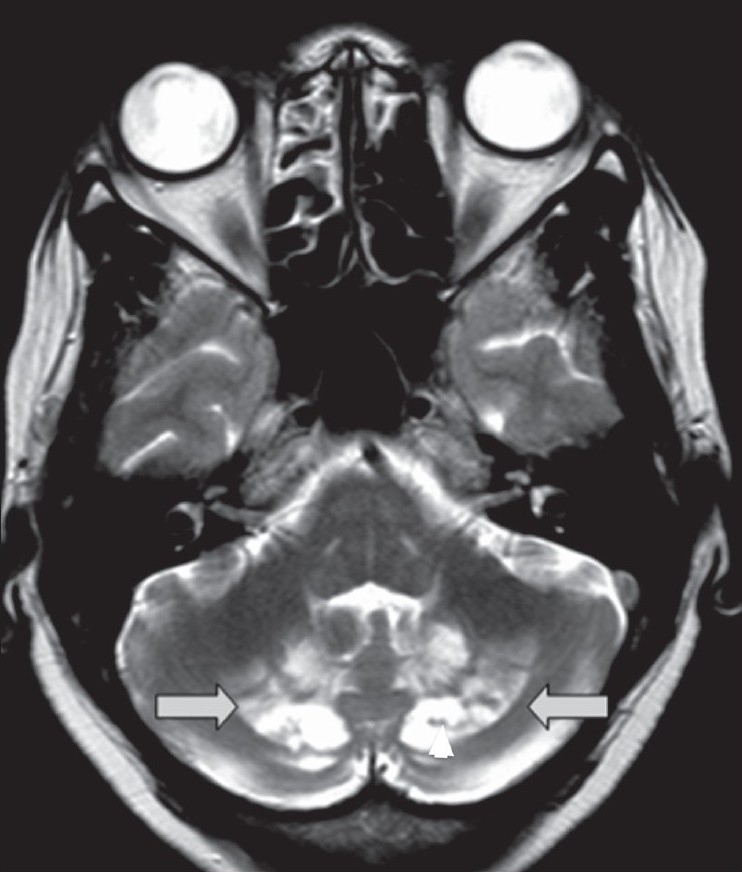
T2W axial MRI image of the brain shows bilateral symmetrical hyperintensities (arrows) involving the dentate nuclei and the deep cerebellar white matter. A few hypointense foci (arrowheads) are seen within the hyperintensities
